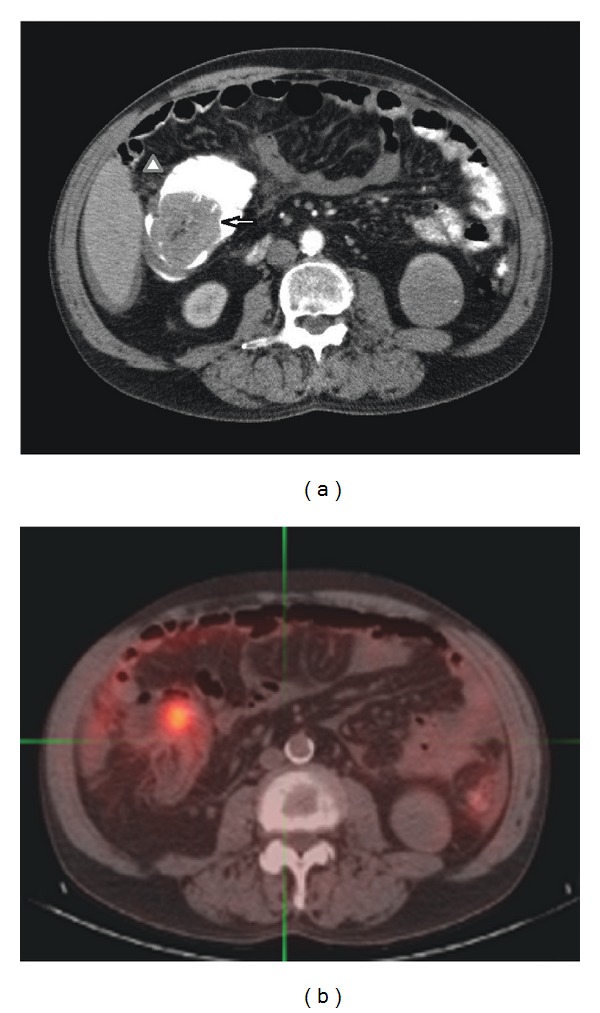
Abdominal imaging. (a) Computed tomography and (b) positron emission tomography scans showed a 5.8 cm partially obstructing cecal mass (arrow) with ascites and peritoneal thickening.
